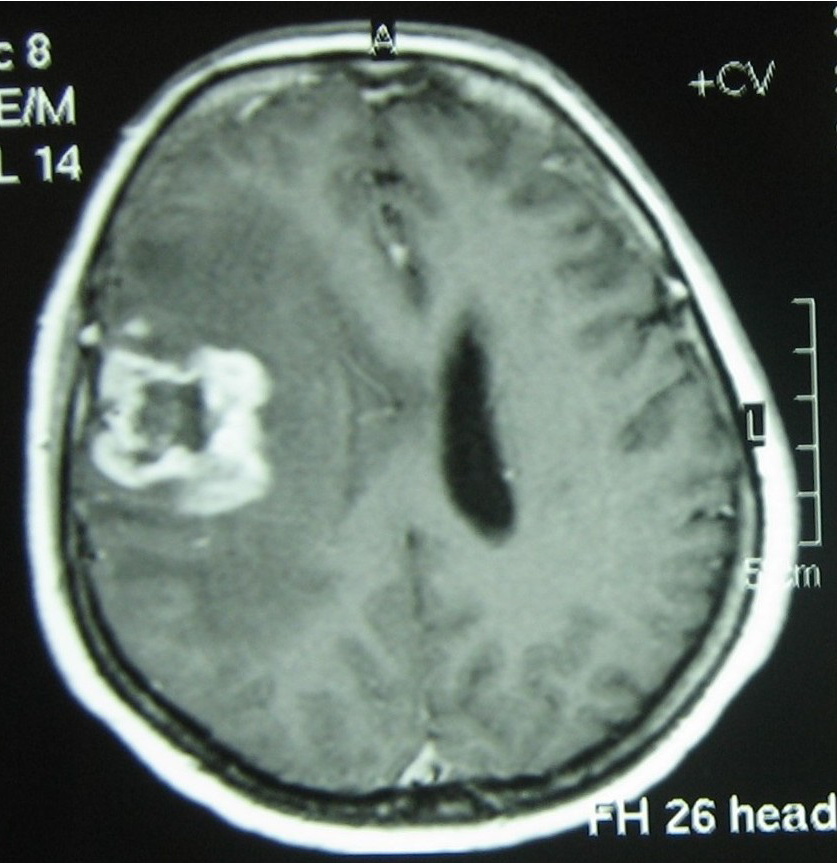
Post contrast magnetic resonance images demonstrates left temporoparietal tumour with heterogenous rim contrast enhancement. On the centre of the tumour, hypointense area indicates nectrotic component of the tumour. There is also obvious compression to lateral ventricular wall with prominent cerebral oedema.
